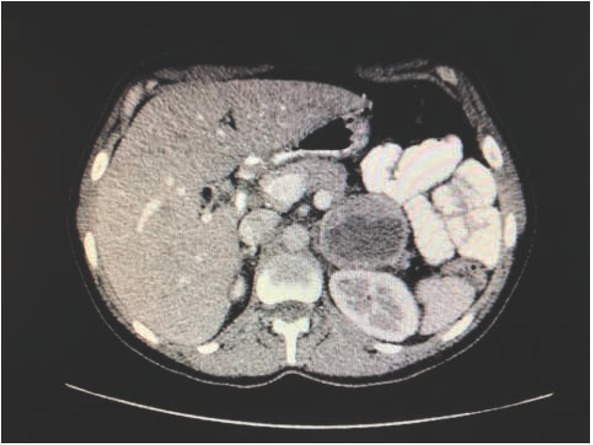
CT scan with tumor resembled pheo of left adrenal gland
